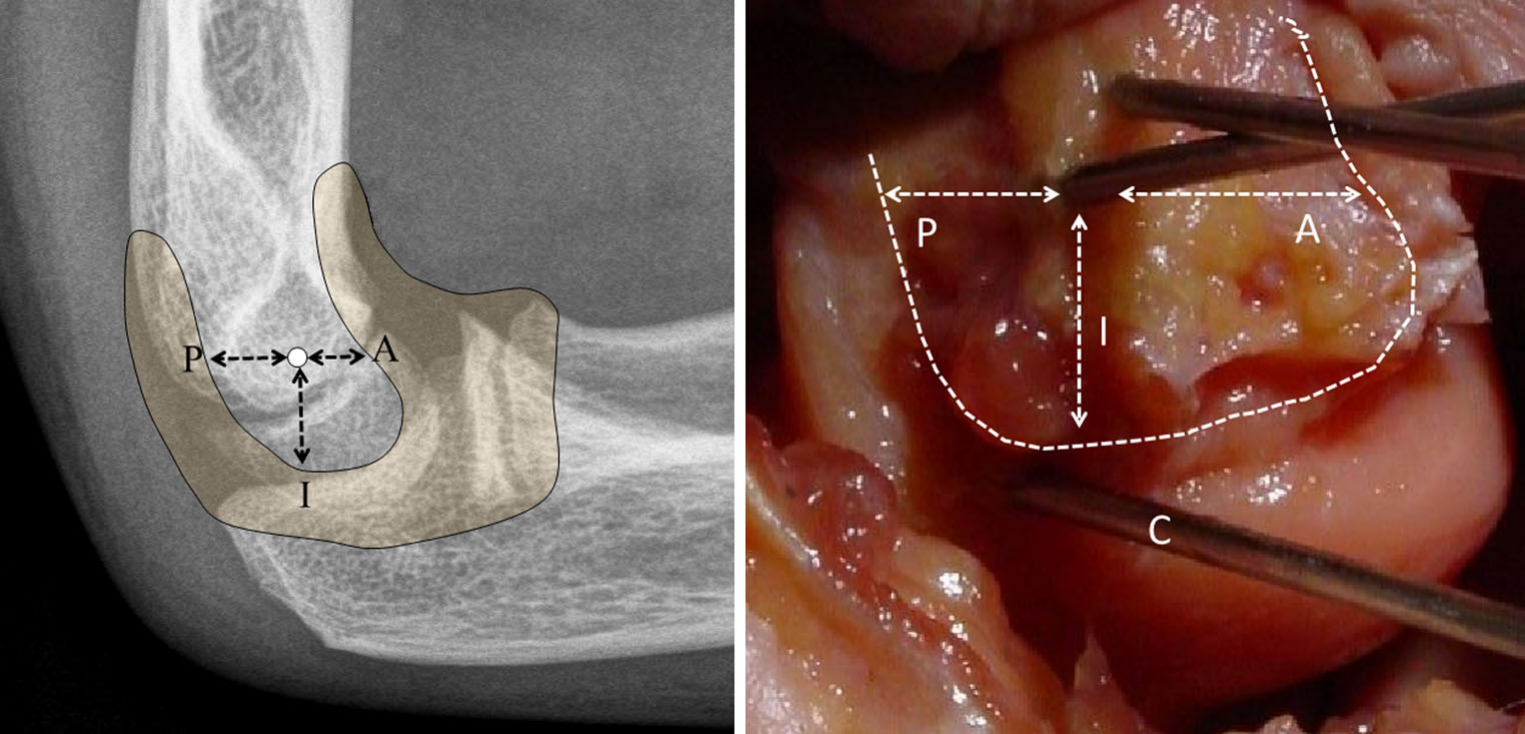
A lateral radiograph and dissection demonstrates capsular reflection and the anterior (*A*), posterior (*P*) and inferior (*I*) distance between the pin and the capsule. *Pin C* is intracapsular, as seen in the dissected elbow
